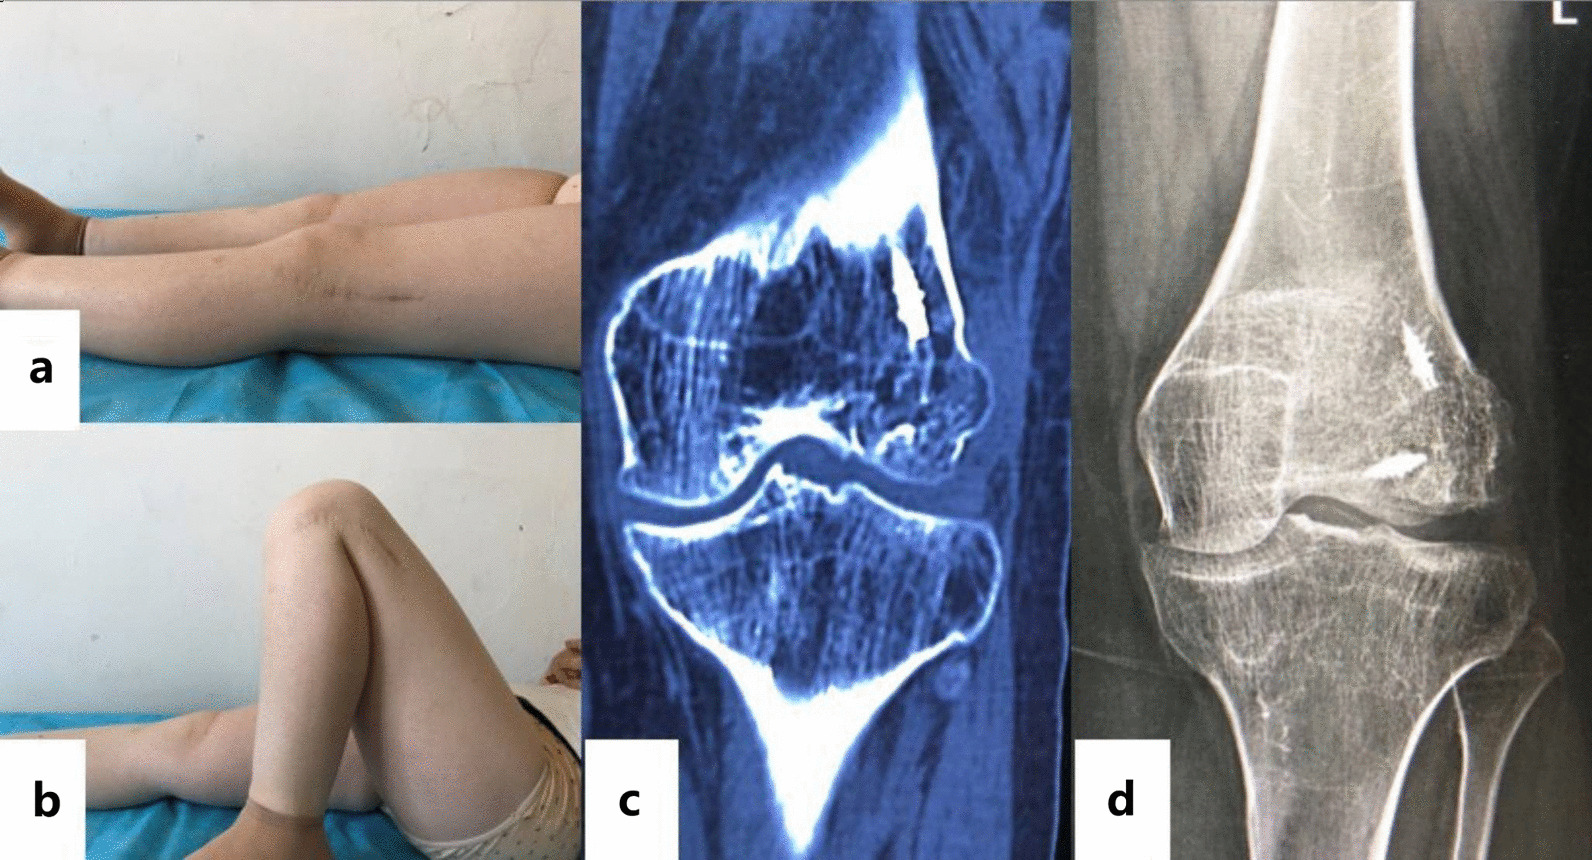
Knee range of motion at 18 months after surgery (a), (b) and CT (c), X-ray (d)
